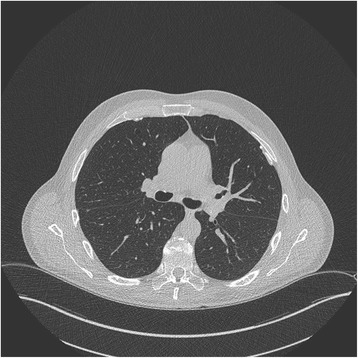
CT-scan of the thorax of 2014 with partially calcified plaque
